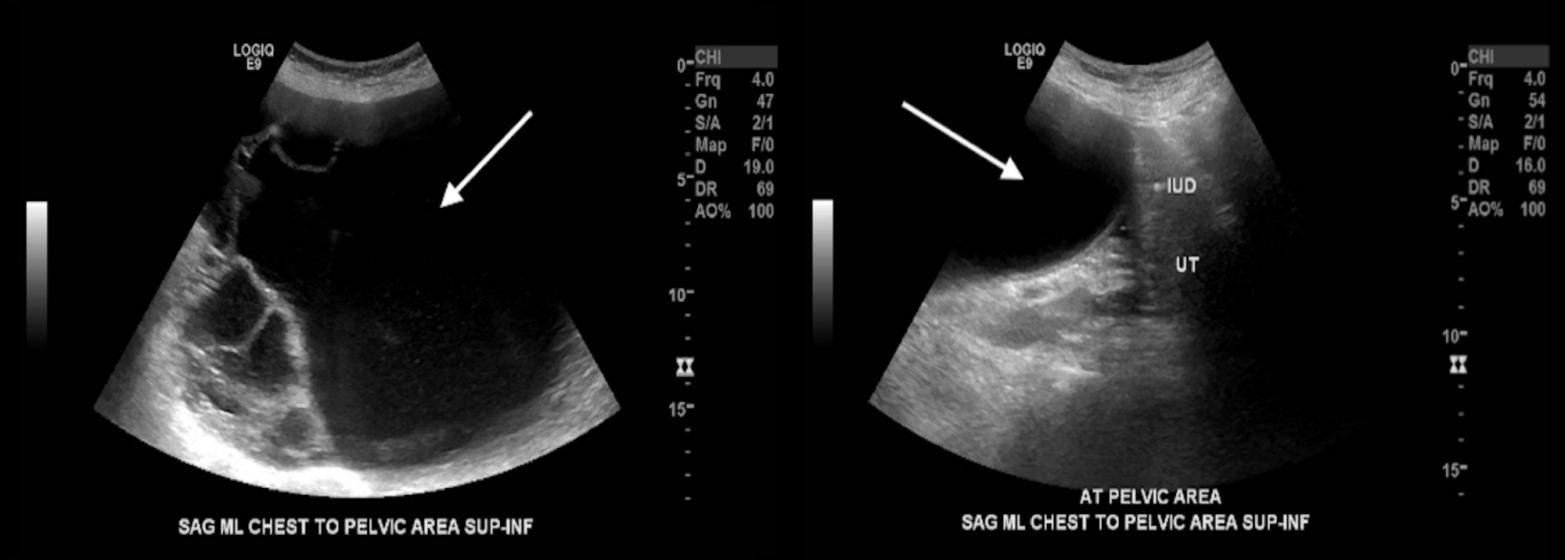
Transabdominal ultrasonography with two sagittal views of the upper abdomen and pelvic area showing a large cystic mass.
